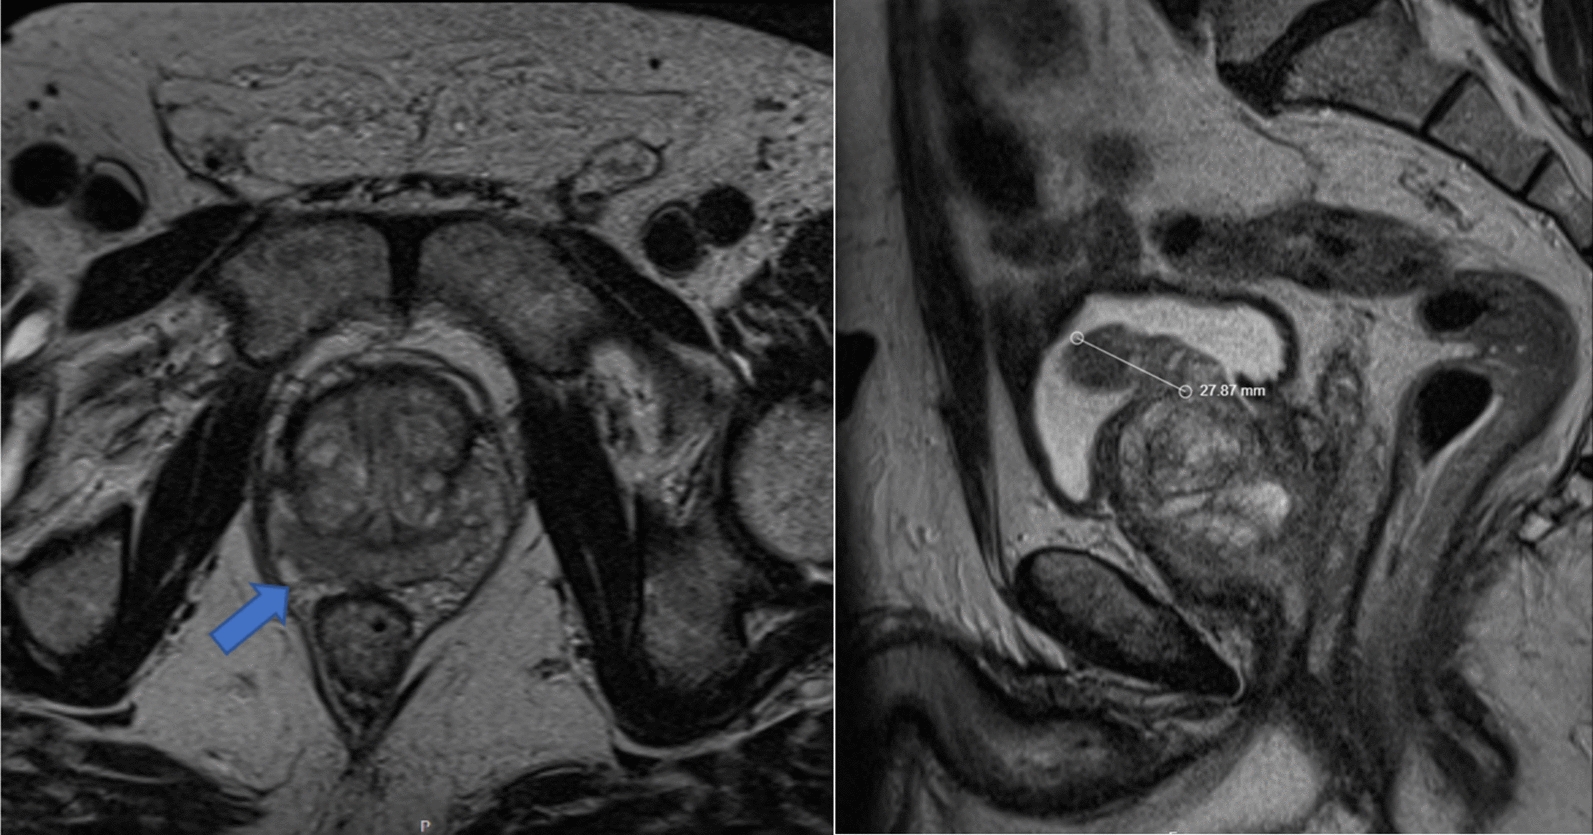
Case 1: Axial slice of multiparametric magnetic resonance imaging demonstrated a prostate imaging reporting and data system 4 lesion concerning for prostate cancer. Sagittal slice of multiparametric magnetic resonance imaging demonstrates an enlarged median lobe
